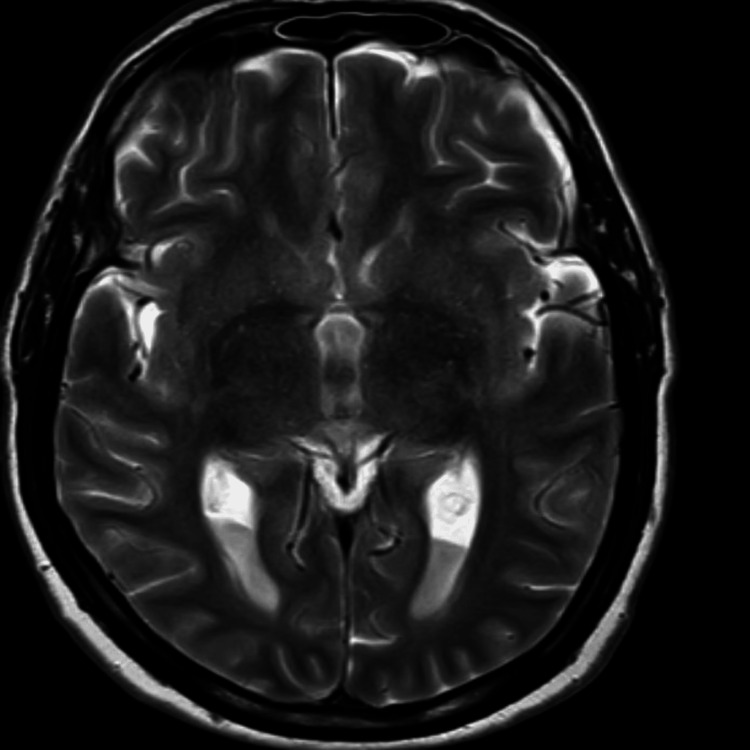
T2-weighted axial image at the level of the thalami demonstrates bilateral symmetrical hyperintensity involving the thalamus and posterior limb of internal capsule, with surrounding white matter also appearing mildly hyperintense
